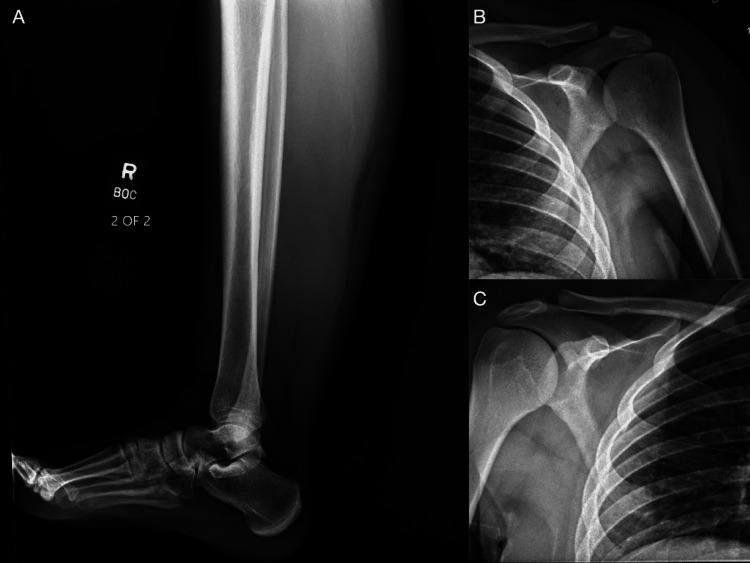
X-ray of tibia and fibula (A), bilateral shoulders (B and C) obtained approximately 3 months since symptom onset. All were unremarkable.
